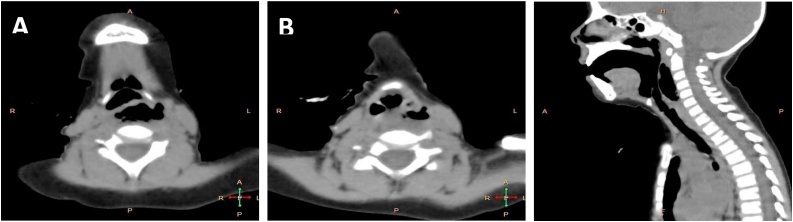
Neck CT; Axial (A,B) and Coronal (C) revealed air retropharyngeal and left parapharyngeal.
